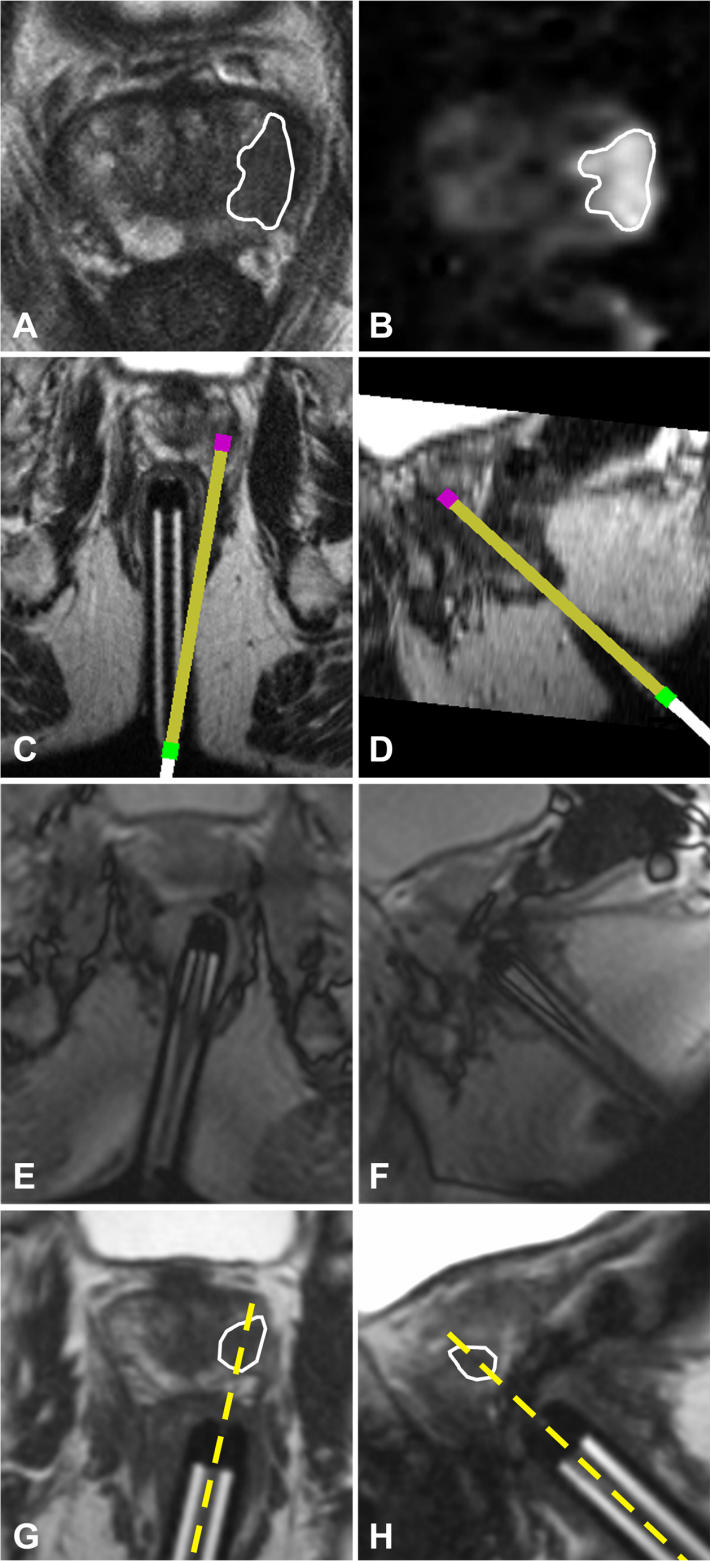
Images in a 63-year-old male with elevated PSA. (a) Axial diagnostic T2-weighted and (b) DW imaging (calculated high b-value image; 1400 s/mm^2^) shows a focal hypointense lesion with diffusion restriction (white outline) suspicious for PCa in the left peripheral zone. (c) Axial and (d) sagittal view of the needle guide trajectory planning. (e) Axial and (f) sagittal BEAT images aligned to the planned trajectory show the final needle guide position after alignment under MR fluoroscopy feedback displayed on the in-room tablet device. (g) Axial and (h) sagittal fast bSSFP scans confirm correct alignment of the needle guide to the CSR in both orientations (yellow dotted lines). Subsequent biopsy showed a Gleason 3 + 4 = 7 prostate cancer
